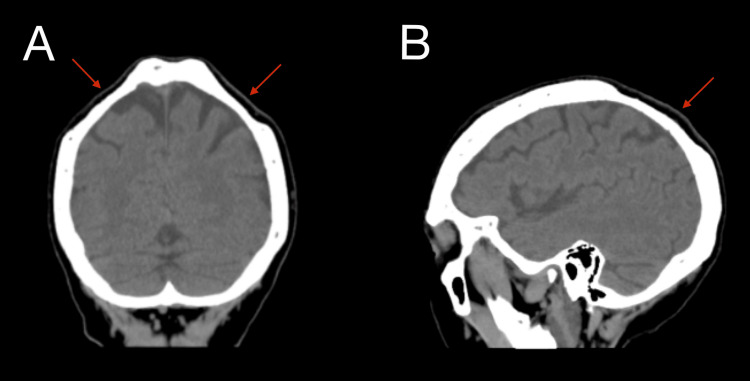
Biparietal osteodystrophy on CT imaging. Coronal (A) and sagittal (B) views demonstrate bilateral thinning of the parietal bone. CT: computed tomography
